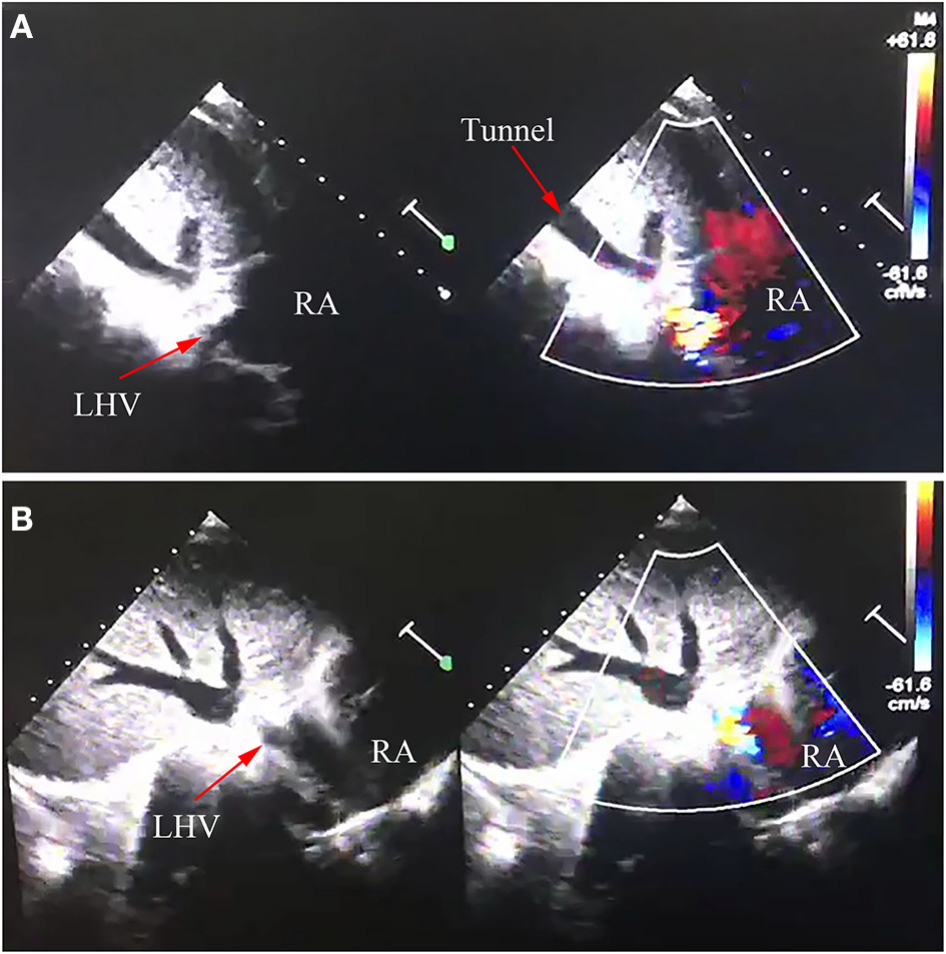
Echocardiography image of anomalous left hepatic venous connection. LHV, left hepatic vein; RA, right atrium. (A,B) Echocardiography image confirmed LHV came into the RA.
